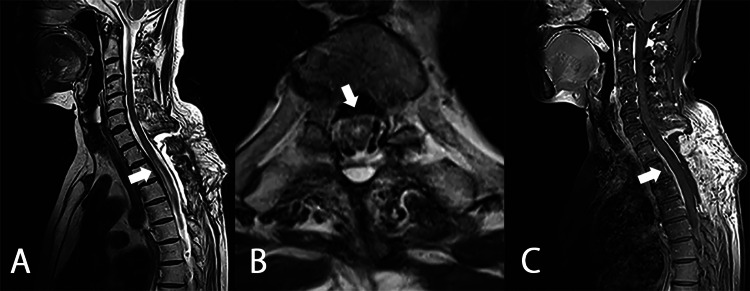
Thoracic MRI of case 1, four weeks after operation and immunosuppressive therapy A: T2WI sagittal; B: T1WI with contrast enhancement axial section; C: T1WI with contrast enhancement sagittal section. The mass has disappeared (white arrows) MRI: magnetic resonance imaging
